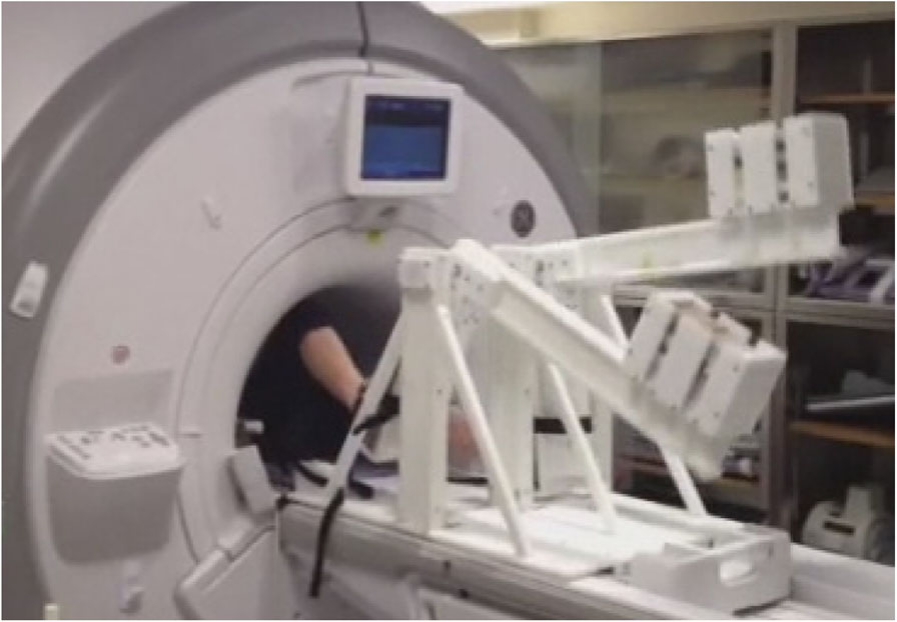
MRI-compatible exercise device. The subject exercises in the magnet bore via a dynamic stepping motion to the beat of a metronome. Resistance is controlled by removable weights at the end of each lever
